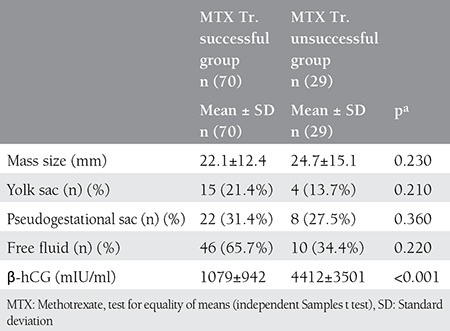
Ultrasonographic and laboratory results of patient groups whose single dose methotrexate treatment was successful and unsuccessful
